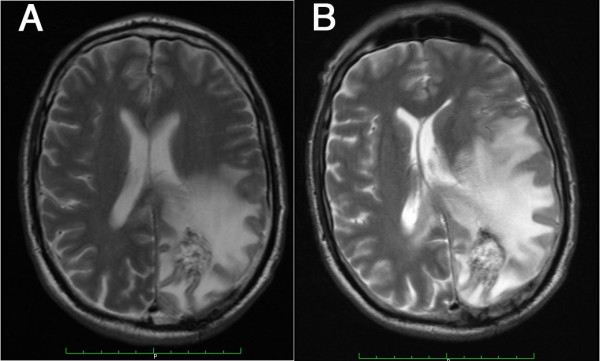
Sulfaslazine-associated peritumor oedema. T2 MRI scans obtained at inclusion, prior to Sulfasalazine treatment in patient #4, and 8 days after the initiation of sulfaslaazine treatment (6 g/day). The images demonstrate an increased peritumoral oedema and the developing midline shift. This patient alo developed severe headaches and withdrew from the study.
